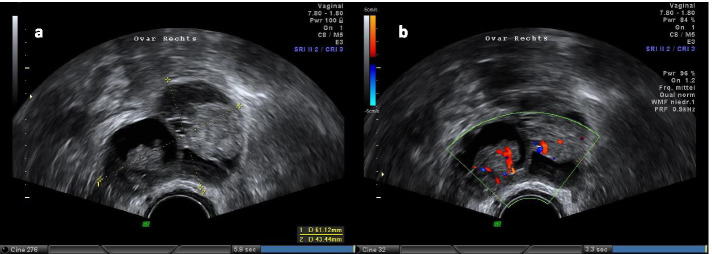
a, b Sonographic presentation at first consultation. At vaginal ultrasound, the right ovary presented highly suspicious, consisting of cystic and solid aspects. a right sided ovarian tumor, b doppler ultrasonography of the ovarian tumor
